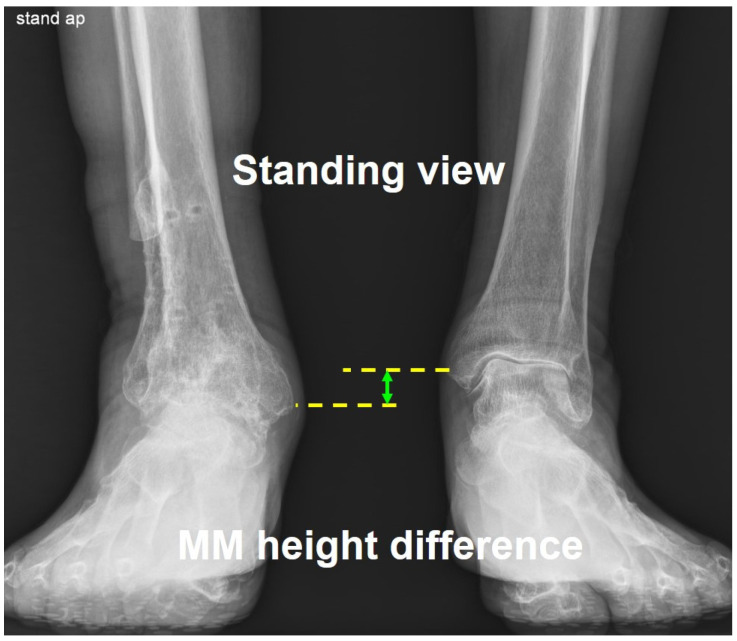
Measurement of the medial malleolar height difference to evaluate the leg length discrepancy. Medial malleolar height difference indicates a difference in height between both medial malleolus in the standing radiograph.
